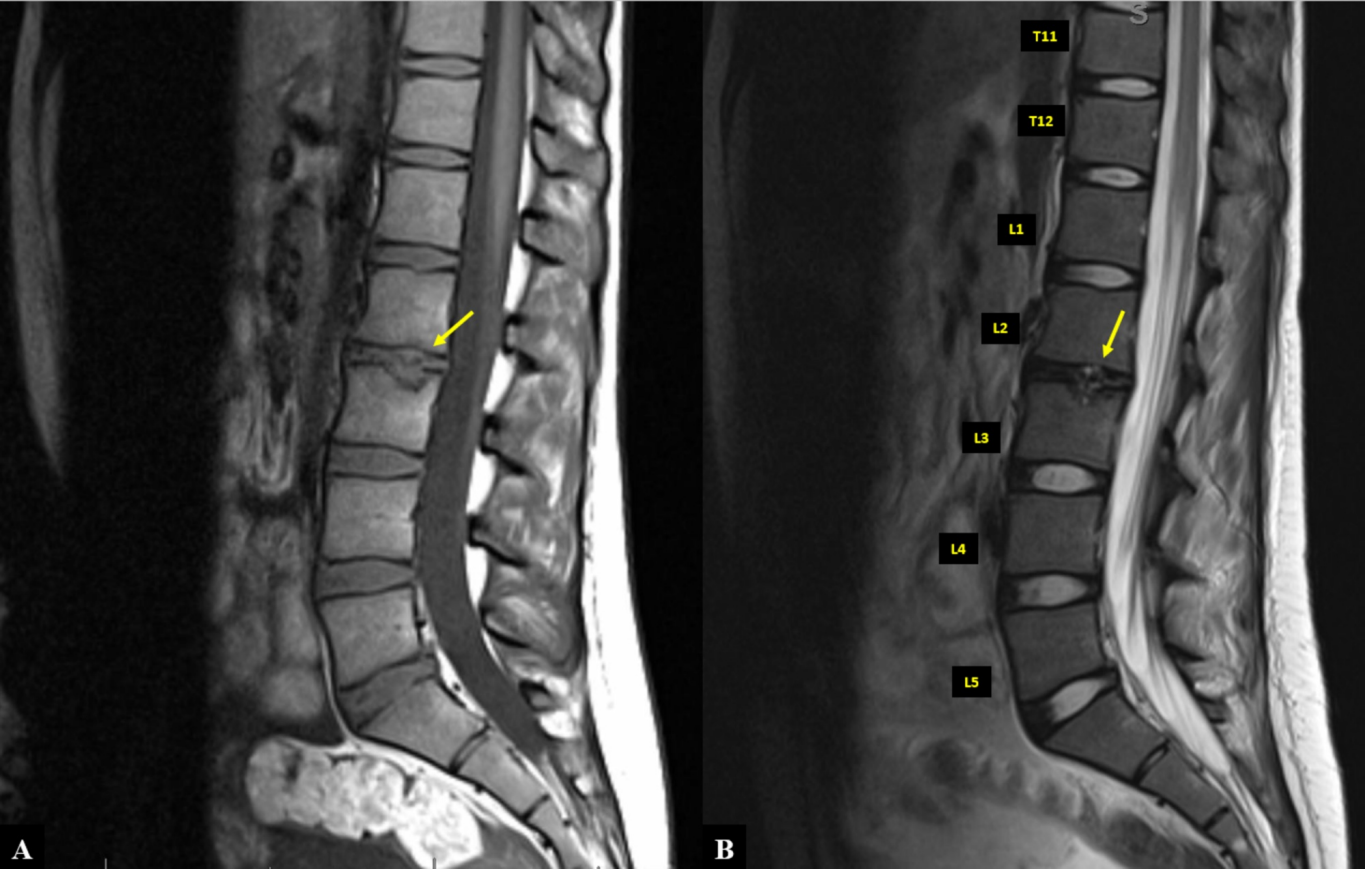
MRI of the lumbar spine A) T1-weighted image. B) T2-weighted image. Arrows indicate the location of the Schmorl's nodule MRI: magnetic resonance imaging
